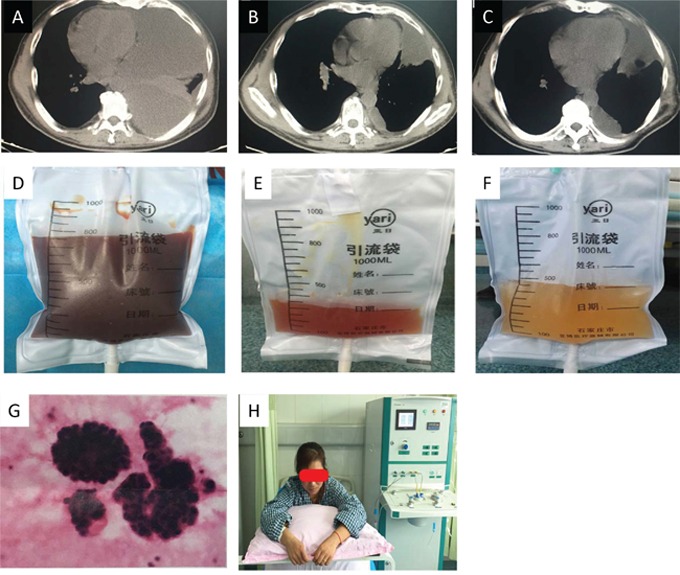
Recurrent pleural effusion after surgical resection of lung adenocarcinoma Panels A, B, C: CT images before HIPEC (A), after one therapeutic course (B), and two therapeutic courses (C), respectively. Panels D, E, F: Color change of the pleural effusion lavage before HIPEC (D), after one therapeutic course (E), and two therapeutic courses (F), respectively. Panel G: Cytologic examination of the lavage fluid before HIPEC demonstrated adenocarcinoma. Panel H: Patient sat up and received HIPEC therapy for one hour.
